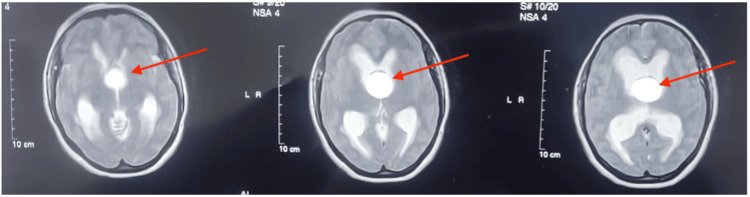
MRI images showing axial T2-weighted sections of the brain There is a large, well-defined, hyperintense cystic lesion (red arrow) located in the anterior superior portion of the third ventricle. The lesion measures approximately 3x3 cm. The surrounding brain structures indicate the presence of moderate hydrocephalus, evidenced by the dilation of the lateral ventricles and transependymal edema. The cerebrospinal fluid appears to be obstructed by the cyst, contributing to the enlargement of the ventricles. The anatomical details suggest that the lesion is consistent with a colloid cyst, though differential diagnoses such as an epidermoid cyst were considered.
